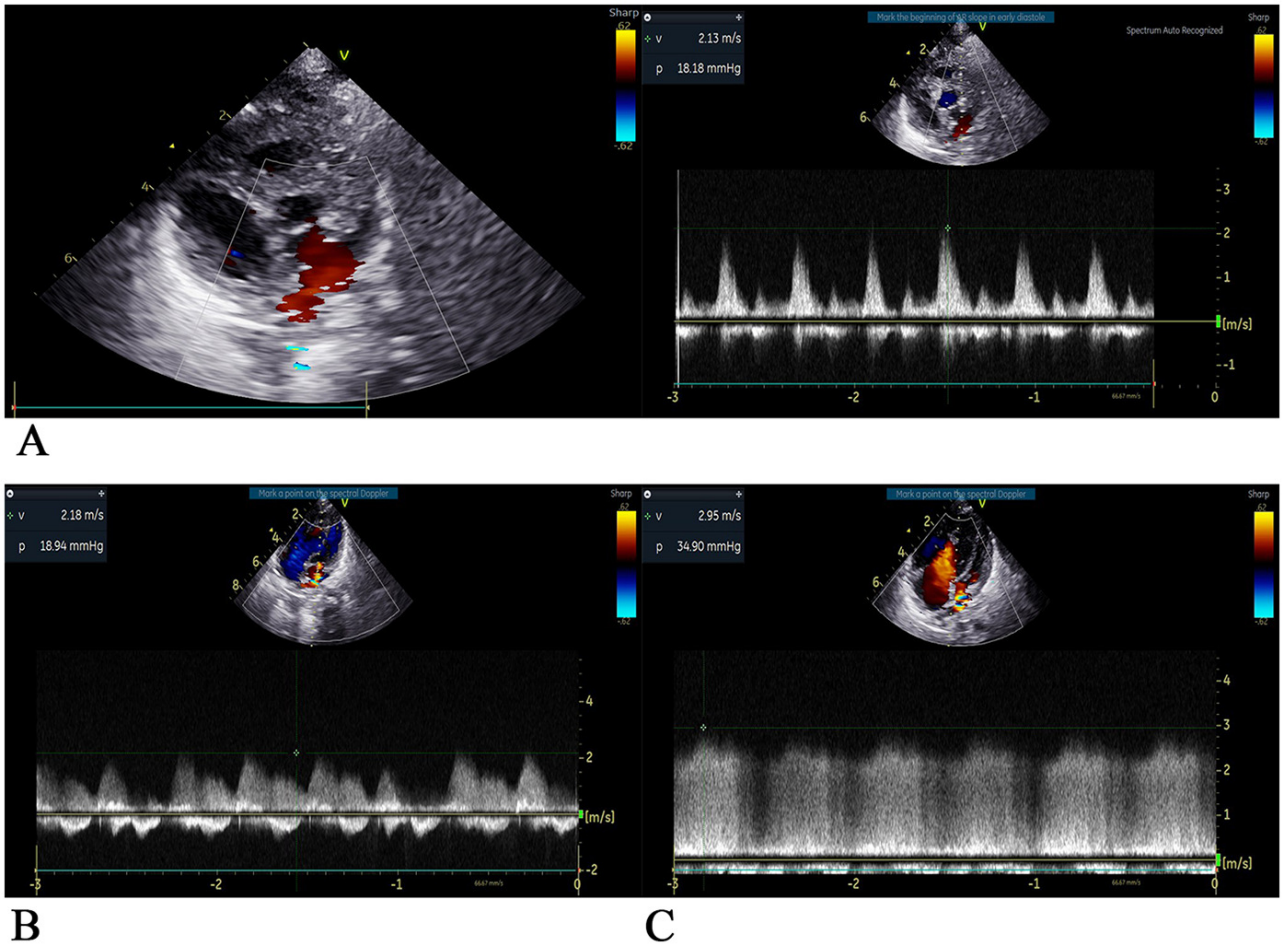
Two-dimensional ultrasound images of the heart at each stage after surgery (A) on the 8th day after surgery, Dopples examination showed that the pulmonary veins were led into the left atrium, the internal diameter of the anastomosis was approximately 5 mm, the forward blood flow velocity of the left atrium of the pulmonary vein was approximately 2.1 m/s, and the pressure difference was 18 mmHg. The estimated pulmonary artery pressure was approximately 53 mmHg based on tricuspid regurgitant flow, indicating fast forward blood flow velocity of the anastomosis and high pulmonary artery pressure. Pericardial effusion (small amount), small regurgitation of the second and tricuspid valves, horizontal shunt of the great artery disappeared, no residual shunt, and residual obstruction were observed. (B) On the 23th day after surgery, ultrasound showed that pulmonary veins were led into the left atrium, the internal diameter of the anastomotic orifice was approximately 3 mm, the forward flow velocity of the anastomotic orifice was fast, the flow velocity was approximately 2.2 m/s, the pressure difference was 19mmHg, the horizontal shunt of the great artery disappeared, no residual shunt and obstruction were observed, atrial septal defect was observed, and the tricuspid valve regurgitant was observed. Pulmonary artery pressure is estimated to be 118 mmHg based on tricuspid regurgitation, pulmonary hypertension (severe). (C) On the 52th day after surgery, ultrasonography showed that the pulmonary veins were led into the left atrium, the internal diameter of the anastomosis was approximately 3.3 mm, the forward blood flow velocity was increased at approximately 3.0 m/s, the pressure difference was 36 mmHg, the atrial septal echo was lost approximately 2 mm, the pulmonary vein opening was small, the pulmonary vein was obstructive, and the tricuspid valve had a large amount of regurgitant flow. Pulmonary artery pressure was estimated to be approximately 100 mmHg based on tricuspid regurgitation, with pulmonary hypertension (severe) and reduced left ventricular systolic function.
